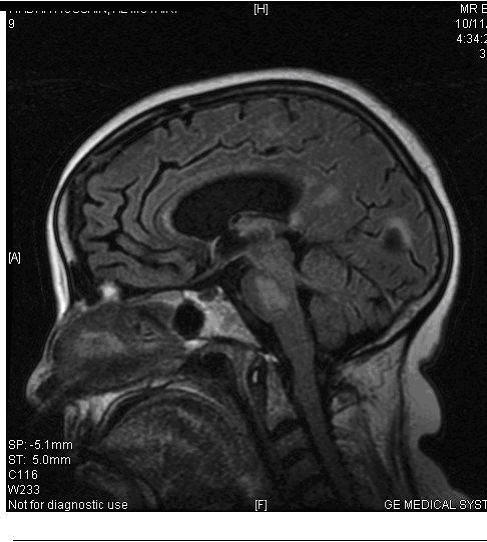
MRI scan of brain showing pontine and extrapontine myelinolysis
